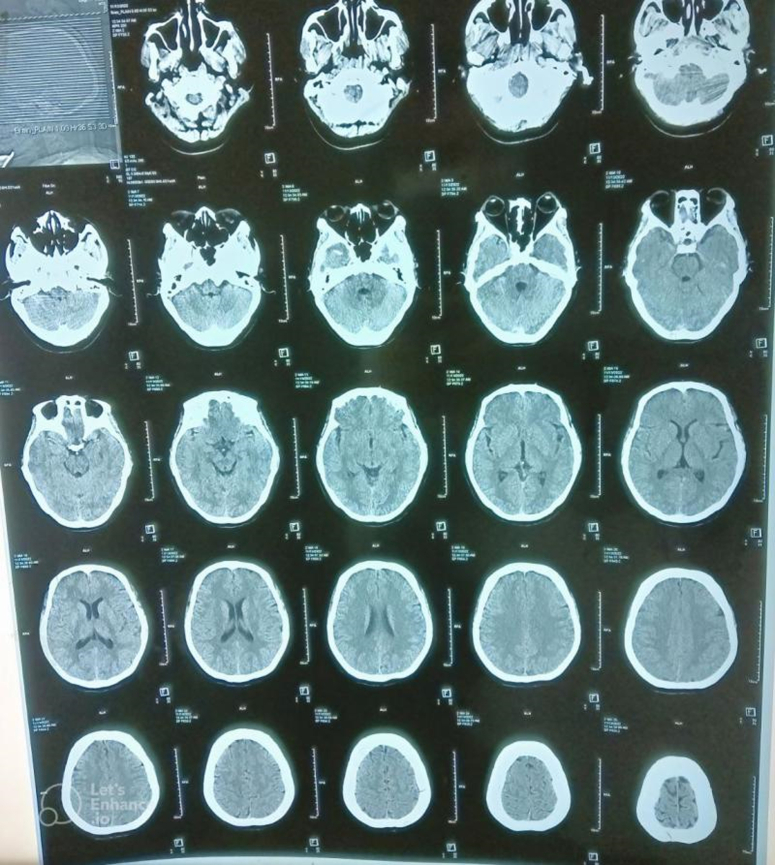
A non-contrast CT of the head.
